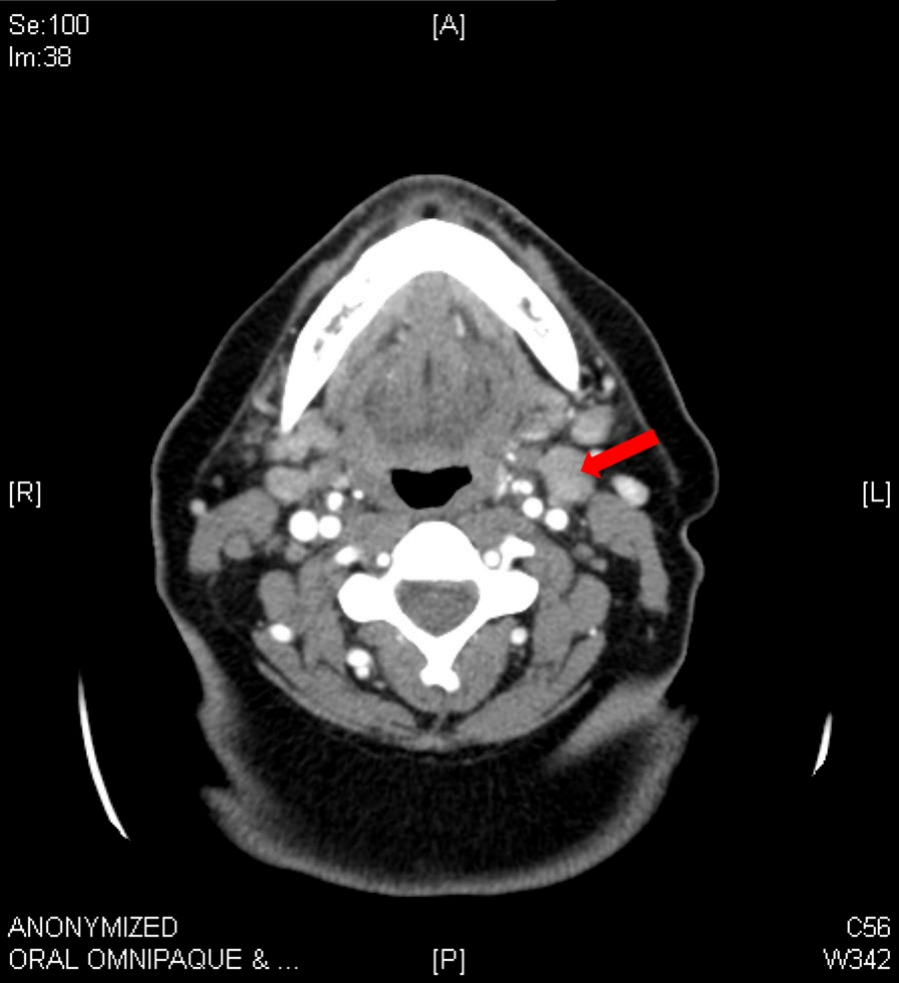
CT of neck demonstrating bilateral diffuse lymphadenopathy with the largest lymph node measuring 2.4 cm (*arrow*)
